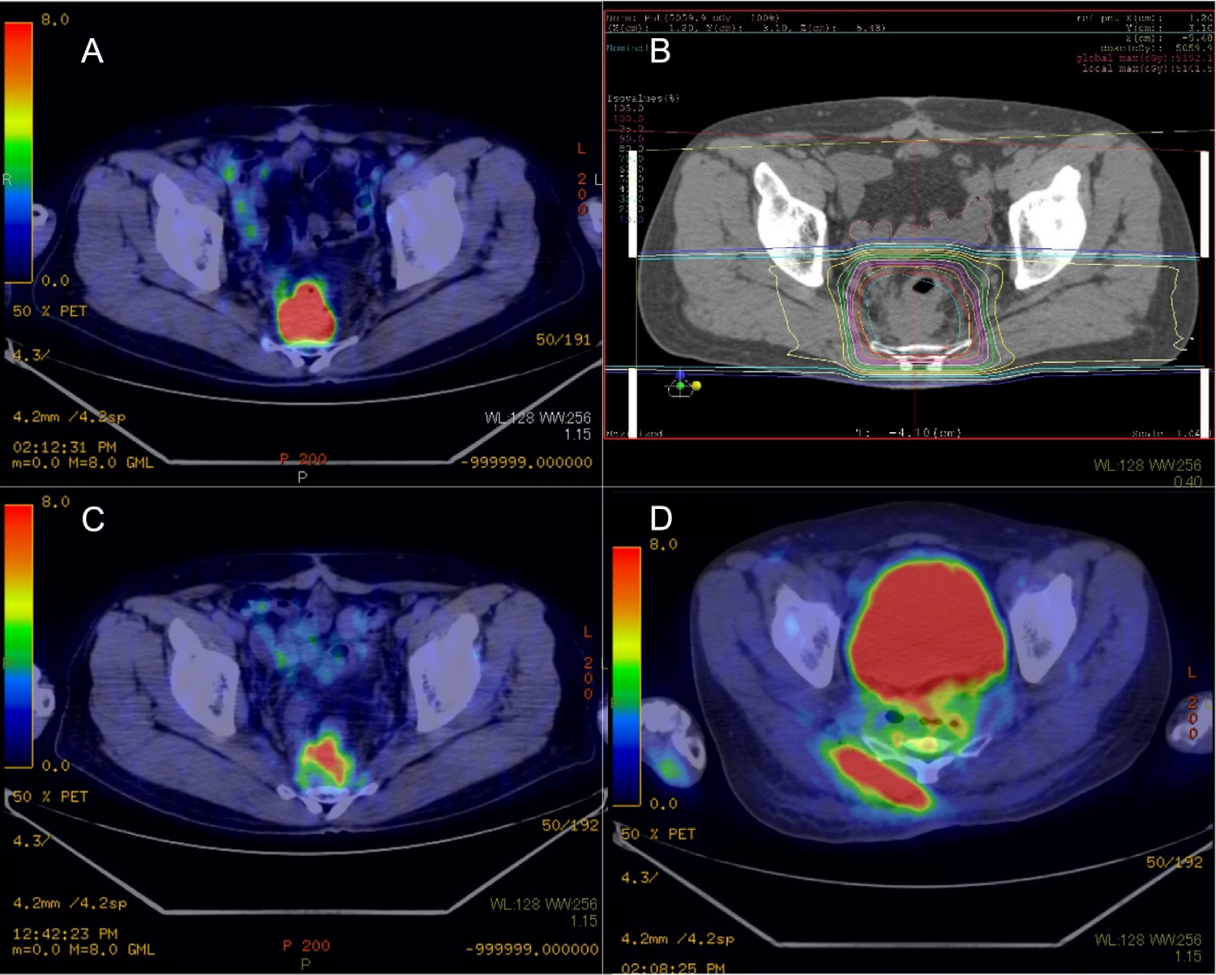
Representative case of a further local recurrence of locally recurrent rectal cancer treated with proton beam therapy (PBT). (A) Image of fluorine-18-fluorodeoxyglucose-positron emission tomography–computed tomography (^18^F-FDG-PET/CT) before PBT. The recurrent tumor showed a high maximum standardized uptake value (SUV_max_ = 22.5). (B) Image of dose distribution of PBT planning. (C) Image of ^18^F-FDG-PET/CT 2 months after PBT. The initial imaging response was a partial response. (D) Image of ^18^F-FDG-PET/CT 11 months after PBT. Further local recurrence occurred in the postsacral region, including the irradiated area.
